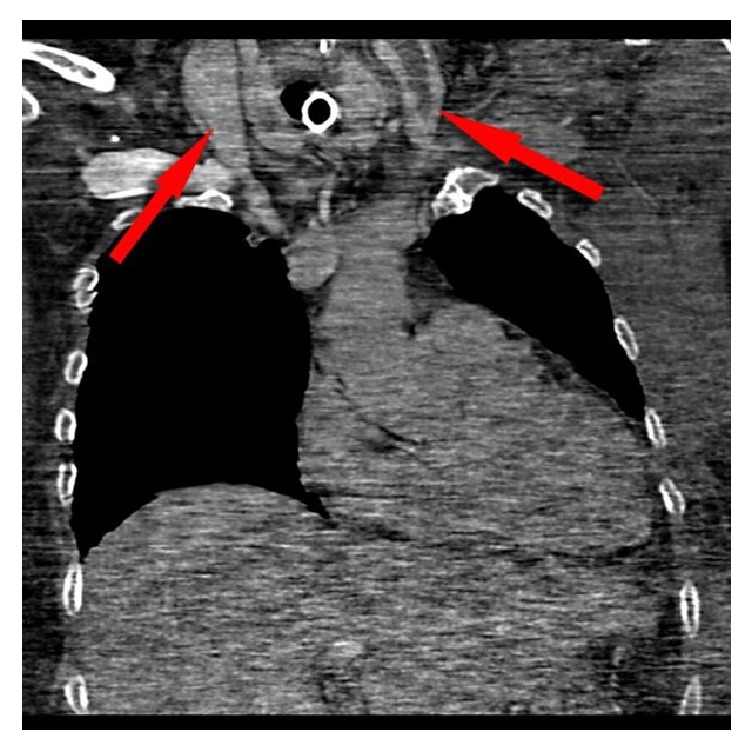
CT scan (coronal image) of the thorax with thrombus adhering to the wall in both internal jugular veins (red arrow).
